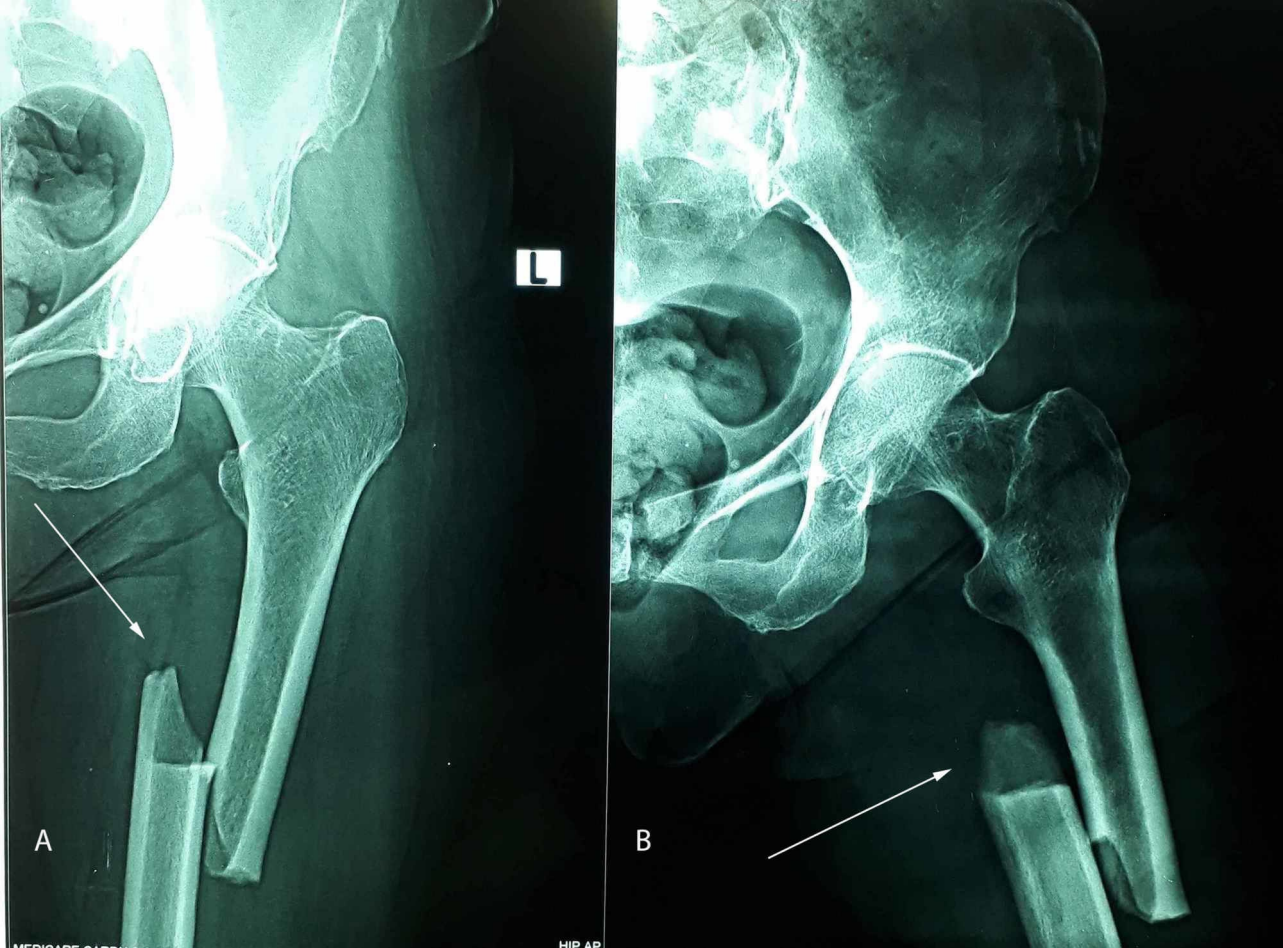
Preoperative anteroposterior (1A) and lateral view (1B) X-ray of a 61-year-old female showing features of atypical diaphyseal femoral fracture
